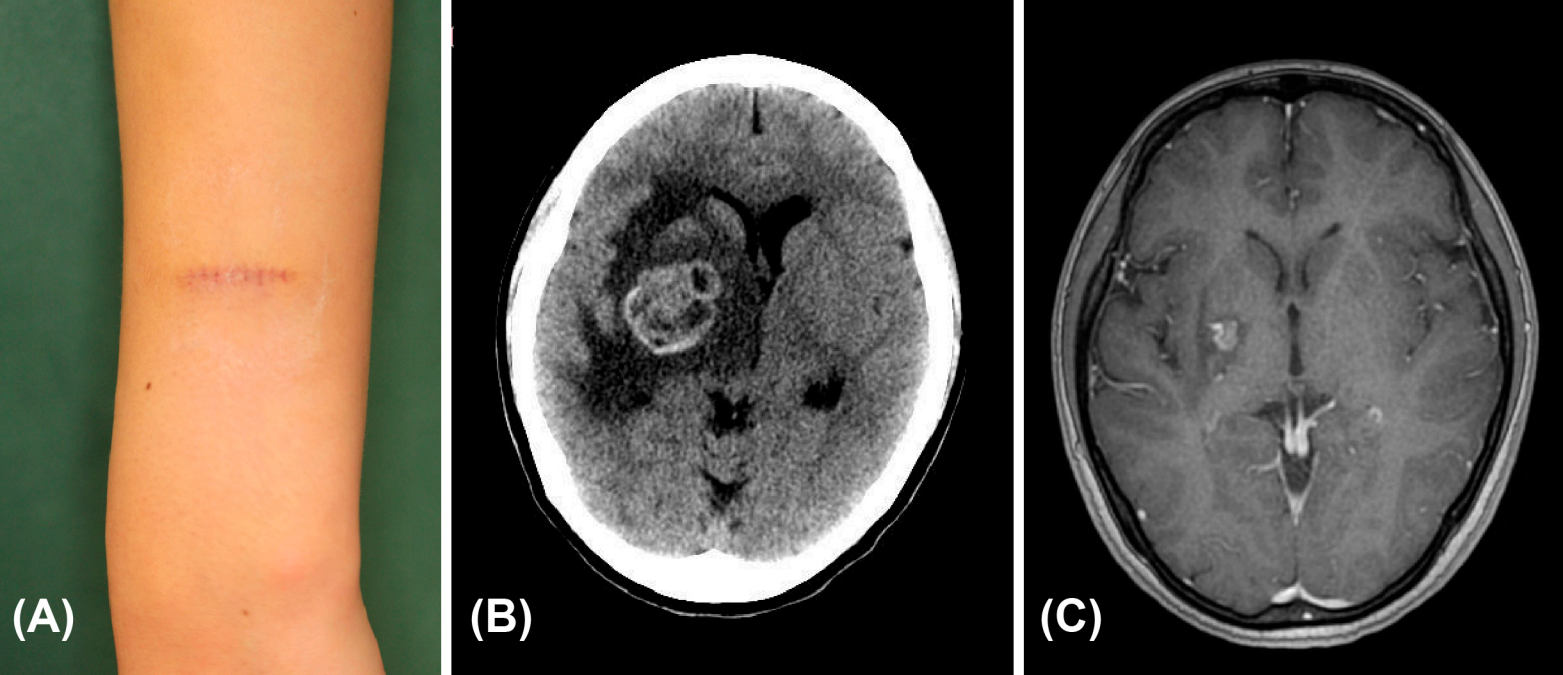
(A) Clinical photograph of Case 2. There were no residual lesions around the scar. (B) Computerized tomography before the treatment with chemotherapy and stereotactic radiotherapy in Case 2. Metastasis measuring 35 mm in diameter is seen in the right basal ganglia. (C) Magnetic resonance imaging 4 years after the treatment with pembrolizumab and stereotactic radiotherapy in Case 2. The size of metastasis has been significantly reduced.
